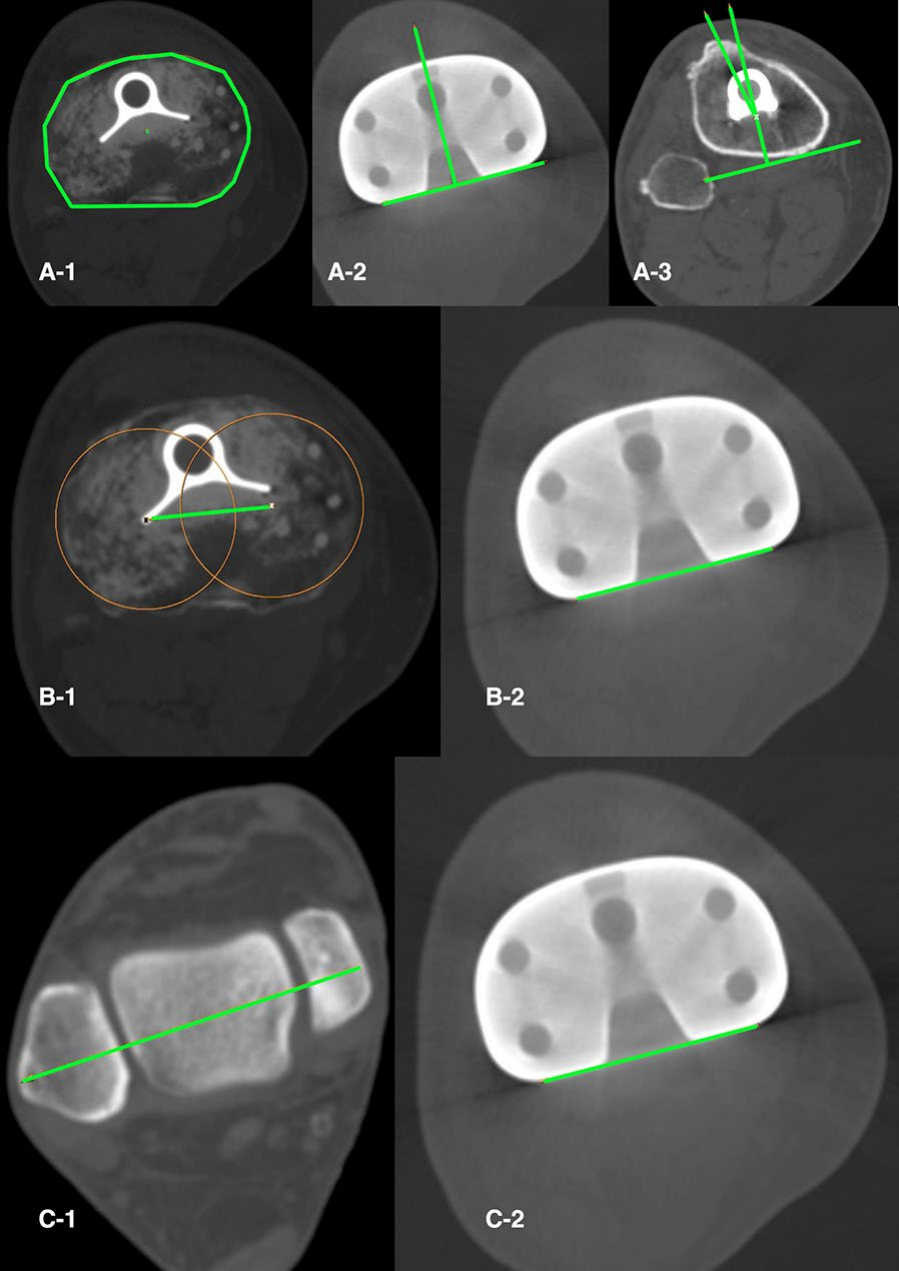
TKA tibia component rotation measures. A Beger’s tibial angle (BTA) formed between the line that connects the geometric centre of the tibial plateau and the tip of the tibial tubercle and the anteroposterior tibial component axis. The geometric centre of the tibial plateau measured in the first CT slice just under the metal tray (A-1) was located and axially transposed to CT slice at the level of the tibial tubercle (A-3). Then, the geometric centre of the tibial plateau and the tip of the tibial tubercle are connected (A-3). The anteroposterior tibial component axis is drowned in a single axial scan through the tibial component perpendicular to the posterior tibial component axis (A-2) and transposed to the CT slice at the level of the tibial tubercle. The posterior tibial component axis was a line tangential to the posterior TKA tibial plateau. The tip of the tibial tubercle is 18° (± 2.6°) externally rotated from the native tibial articular surface, the tibial component was considered neutral (0°) when internally rotated 18° in relation to the tip of the tibial tuberosity. B Anatomic tibial angle (ATA) formed between the anatomic tibial axis (B-1) and the posterior tibial component axis (B-2). The anatomic tibial axis is the line defined by the geometric centre of the lateral tibial plateau and the geometric centre of medial tibial plateau measured in the first tibial CT slice just under the TKA tibial component (B-1). C Bimalleolar posterior tibial component angle (BM_PTCA) is the angle formed by the transmalleolar axis (C-1) between the centre of the medial malleolus and the centre of the fibula in an axial slice situated at the ankle centre and bisecting the malleoli and the posterior tibial component axis (C-2)
